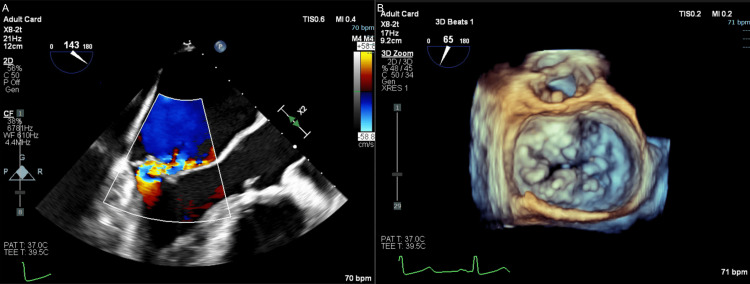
Preoperative transesophageal echocardiography (TEE).
